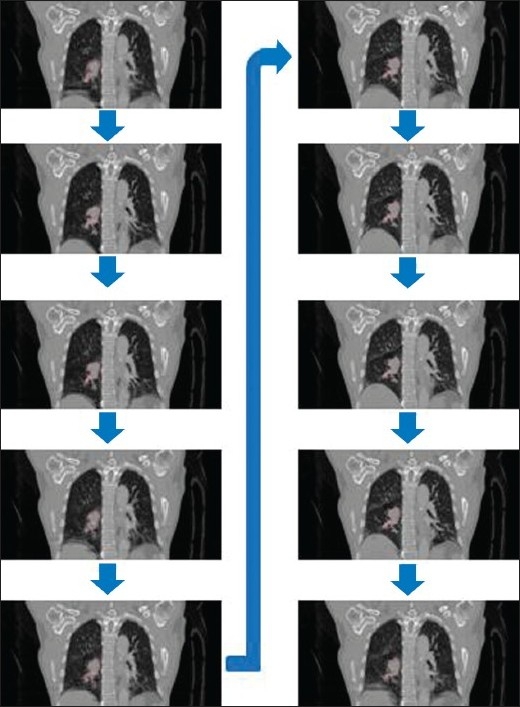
Coronal slices depicting the peak-inhale phase (phase 1, top left), peak-exhale phases (phases 5-6, bottom left and top right), peak-inhale phase (phase 10, bottom right) and a few others of a 4-D computed tomography (4D CT) scan. The contour represents the tumor volume as delineated by the physician on the planning CT
